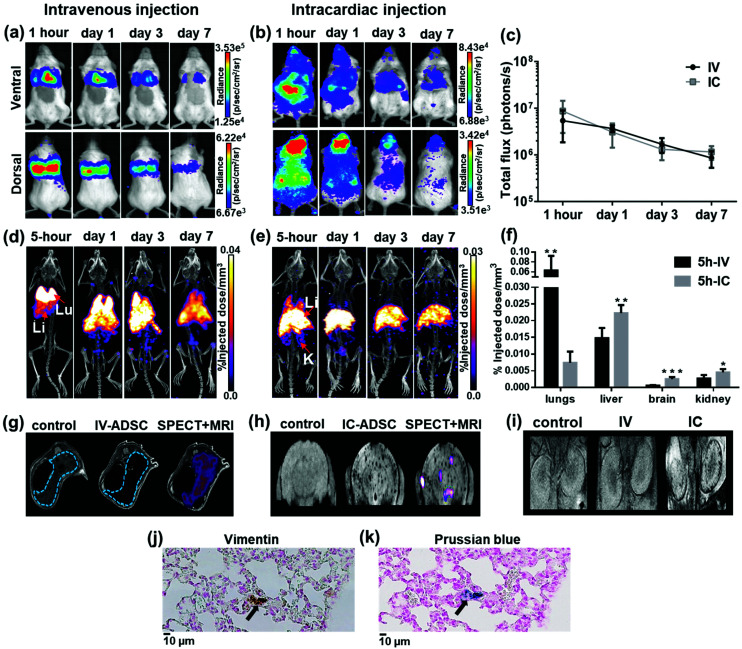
Whole body distribution of ^111^In-modified-SPION labelled ADSCs following IV or IC injection at different time points. (a and b) BLI signal in the chest and the whole body decreased over time. (c) BLI signal from the whole body decreased over time (photons per s in log scale). (d & e) Decay corrected SPECT/CT images of dual labelled ADSCs uptake in organs after IV and IC injection (labels: Lu = lungs, Li = liver, S = spleen and K = kidney). (f) 3D ROI quantification of SPECT signal in lungs, liver, brain and kidney at 5-hour after IV & IC injection calculated as %ID mm^–3^ after decay correction (IV *vs.* IC  *P* = 0.0027 in lung,  *P* = 0.0025 in liver, * *P* = 0.0001 in brain & * *P* = 0.0217 in kidney as measured with unpaired two-tailed *t* test). (g & h)
